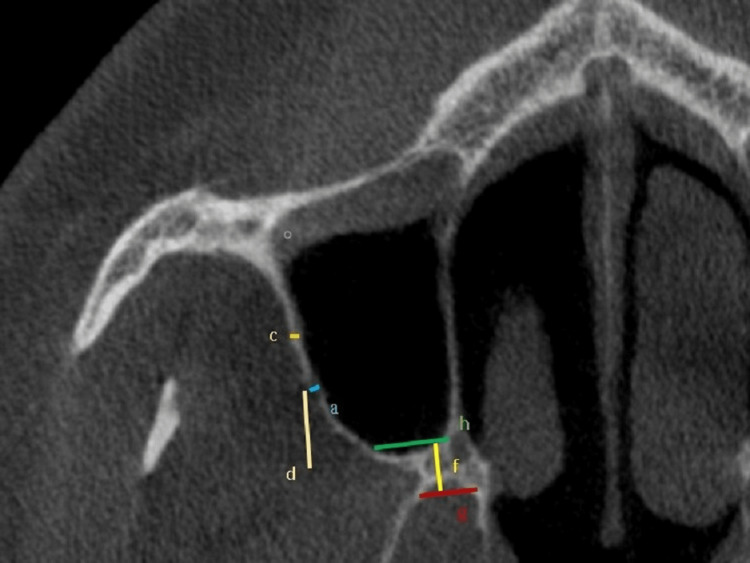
AAA diameter and other measurements made on CBCT. a = AAA diameter; c = thickness of the lateral sinus wall; d = distance from the AAA to the alveolar crest; f = height of the residual alveolar ridge; g = width of the residual alveolar ridge (basal level); h = width of the residual alveolar ridge (crest level). AAA = alveolar antral artery; CBCT = cone-beam computed tomography
